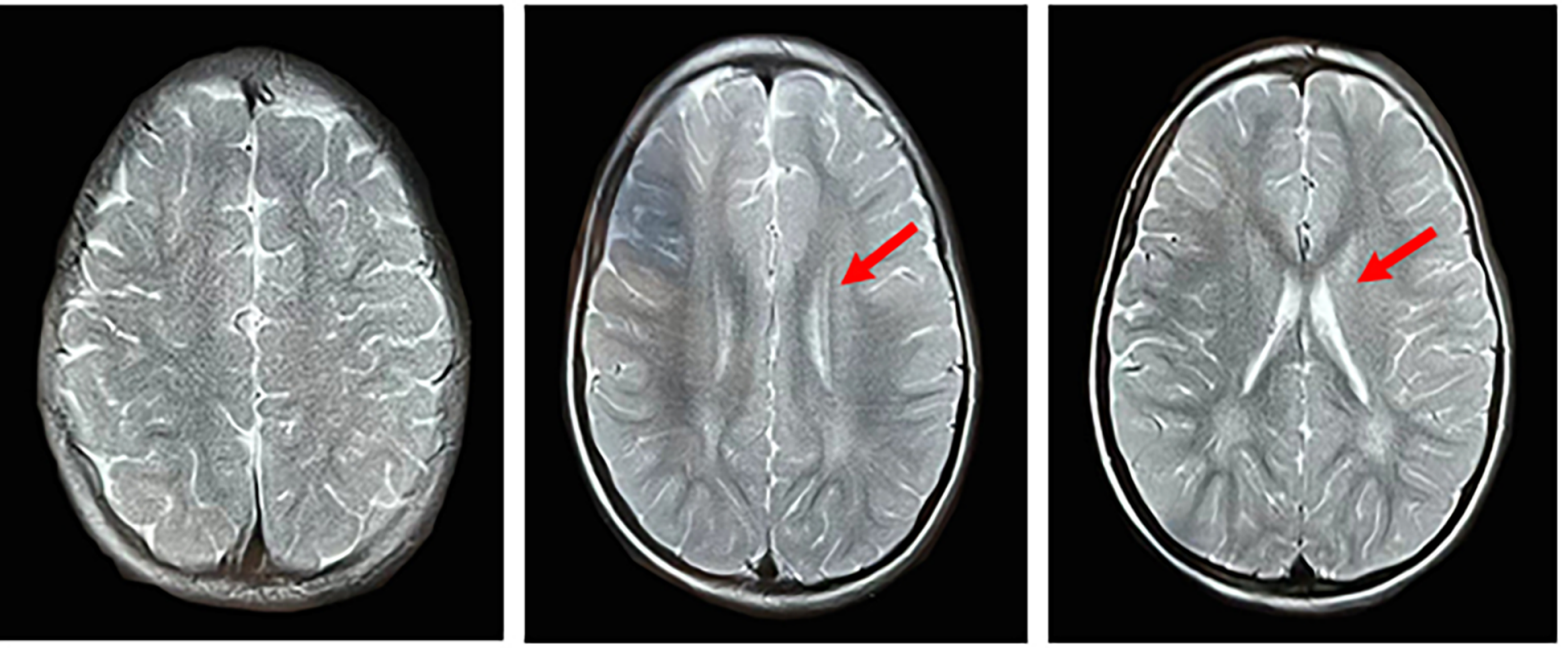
MRI image of the proband. It indicates symmetrical abnormal signals in the white matter area around the bilateral ventricles, mainly around the occipital horns. The lesions showed long T1, long T2 signals and FLAIR high signals.
